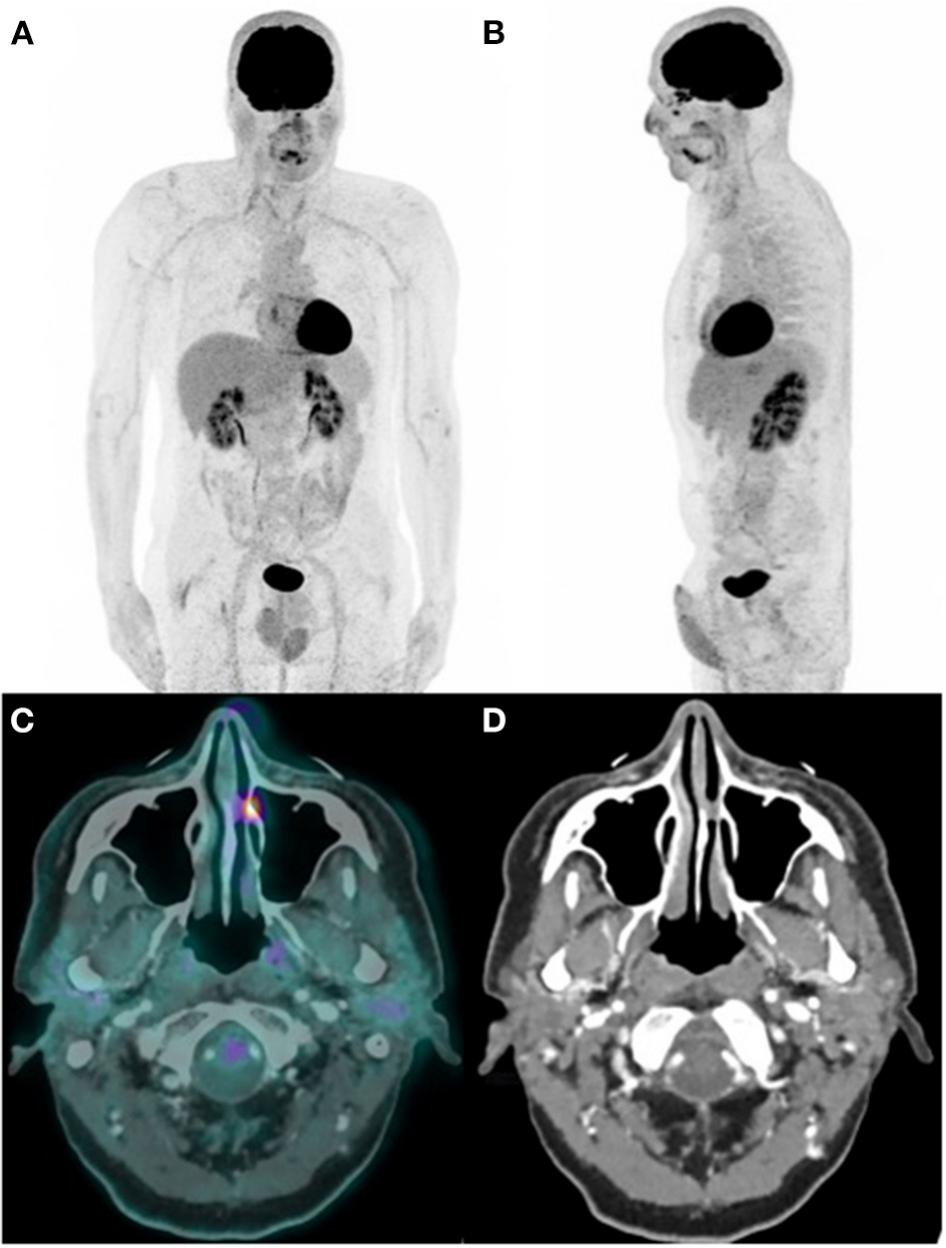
Fluoro-D-glucose PET/CT (FDG-PET/CT) images. (A,B) PET MIP (C) fused PET/CT, and (D) CT.
